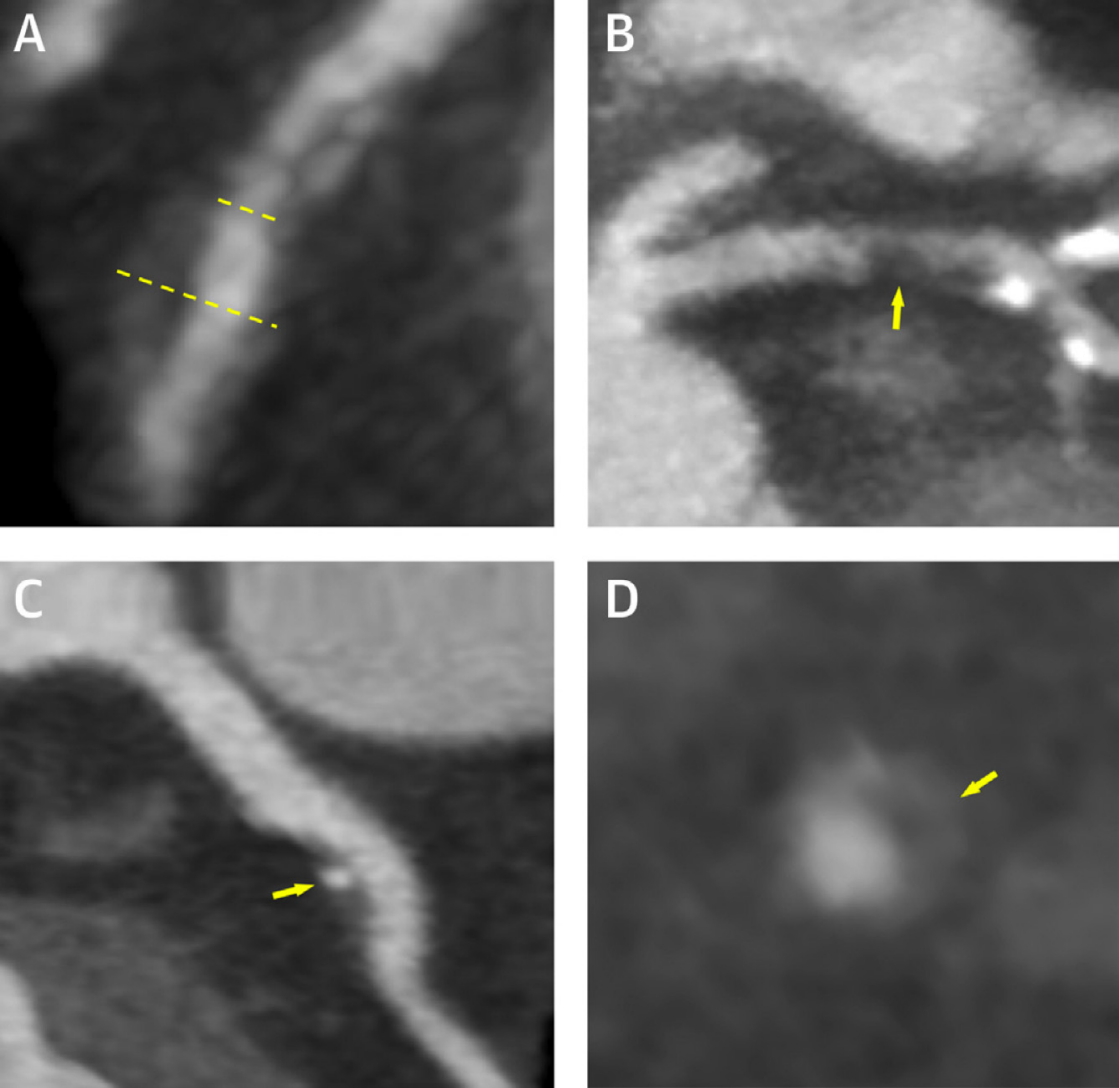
High-risk plaque features on CT coronary angiography. Features of high-risk atherosclerotic plaque including (A) positive remodelling, (B) low attenuation plaque, (C) spotty calcification and (D) the ‘napkin ring’ sign. Images courtesy of Williams et al.^7^
